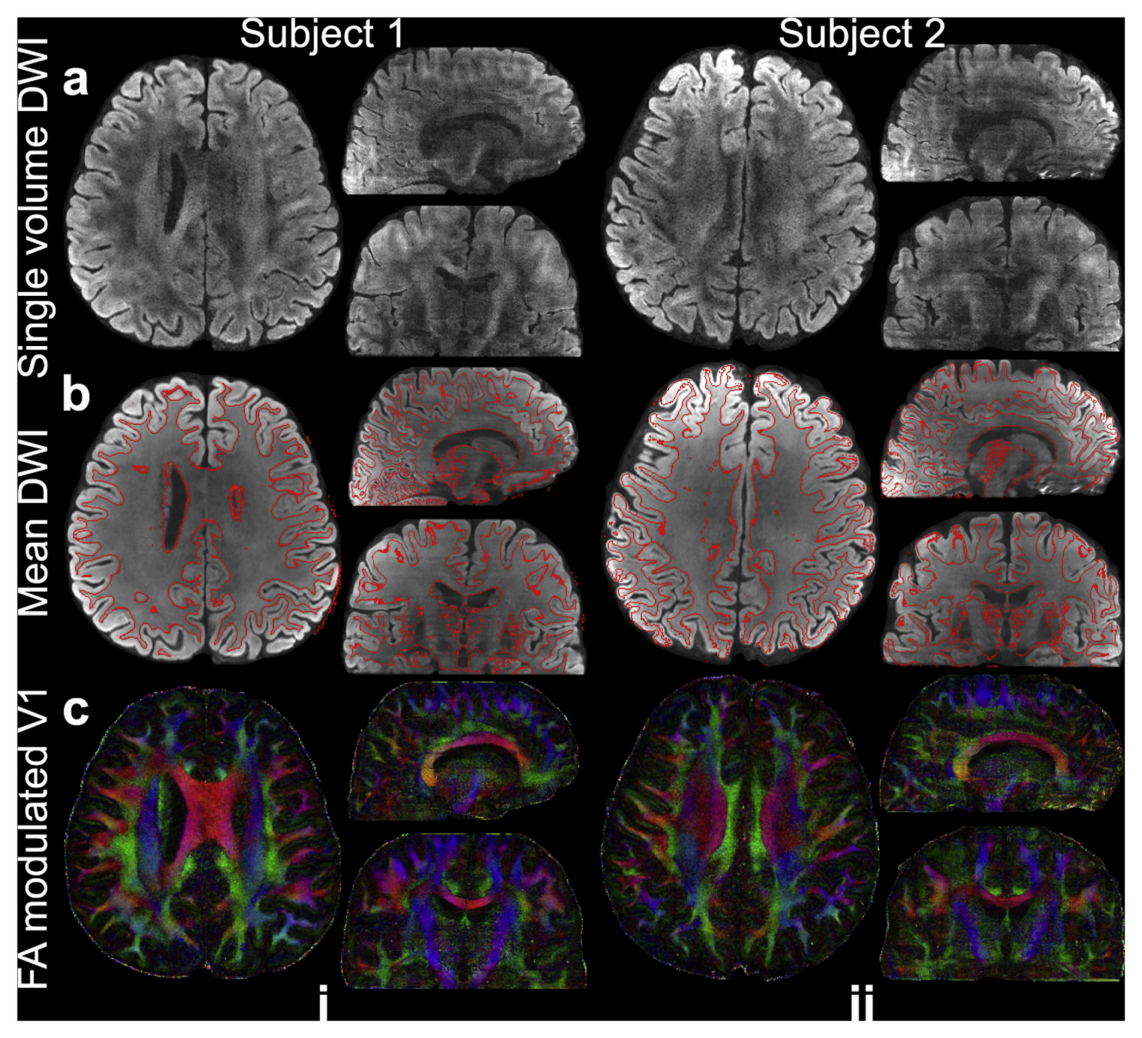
Data from 3T 0.53 mm protocol. (a) In-vivo diffusion data of two subjects (*b* = 1000 s/mm^2^) including diffusion-weighted image (DWI) along (0.5, –0.86, 0.02), (b) 20-direction mean DWI with gray-white matter boundary derived from the T1w image using FSL’s “fast” overlayed, (c) FA modulated V1 at 0.53 mm isotropic resolution.
